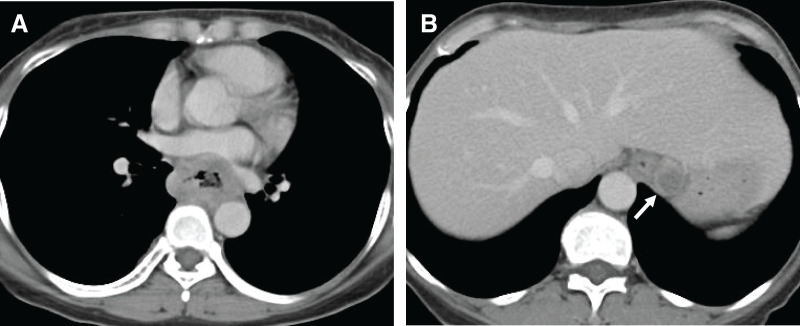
CT images. (A) A large tumor in the middle thoracic esophagus compresses the heart; invasion of the aorta is suspected. (B) A swollen left paracardial lymph node (arrow).
